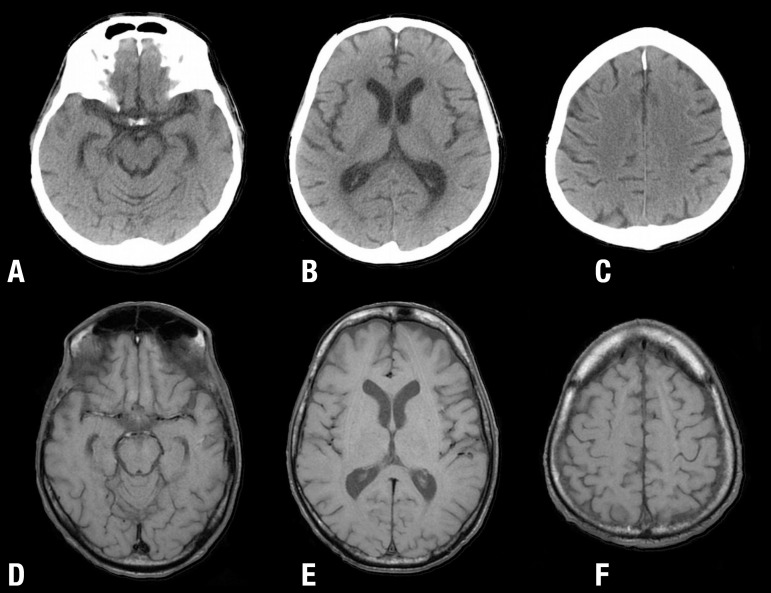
[A, B, C] Conventional CT and [D, E, F] MRI T1W images of two different
